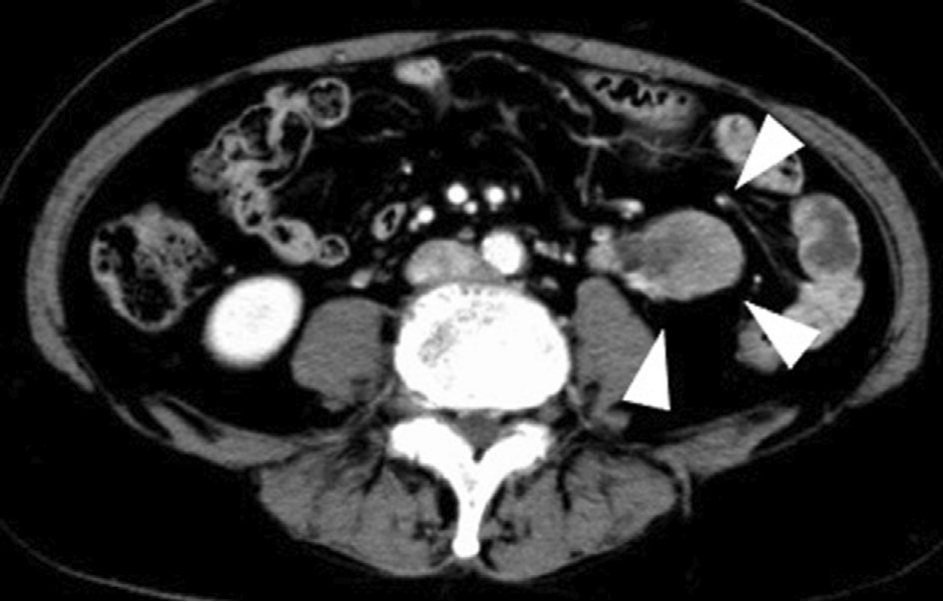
Pre‐treatment imaging. Abdominal contrast‐enhanced CT revealed a left ureteral tumor.
